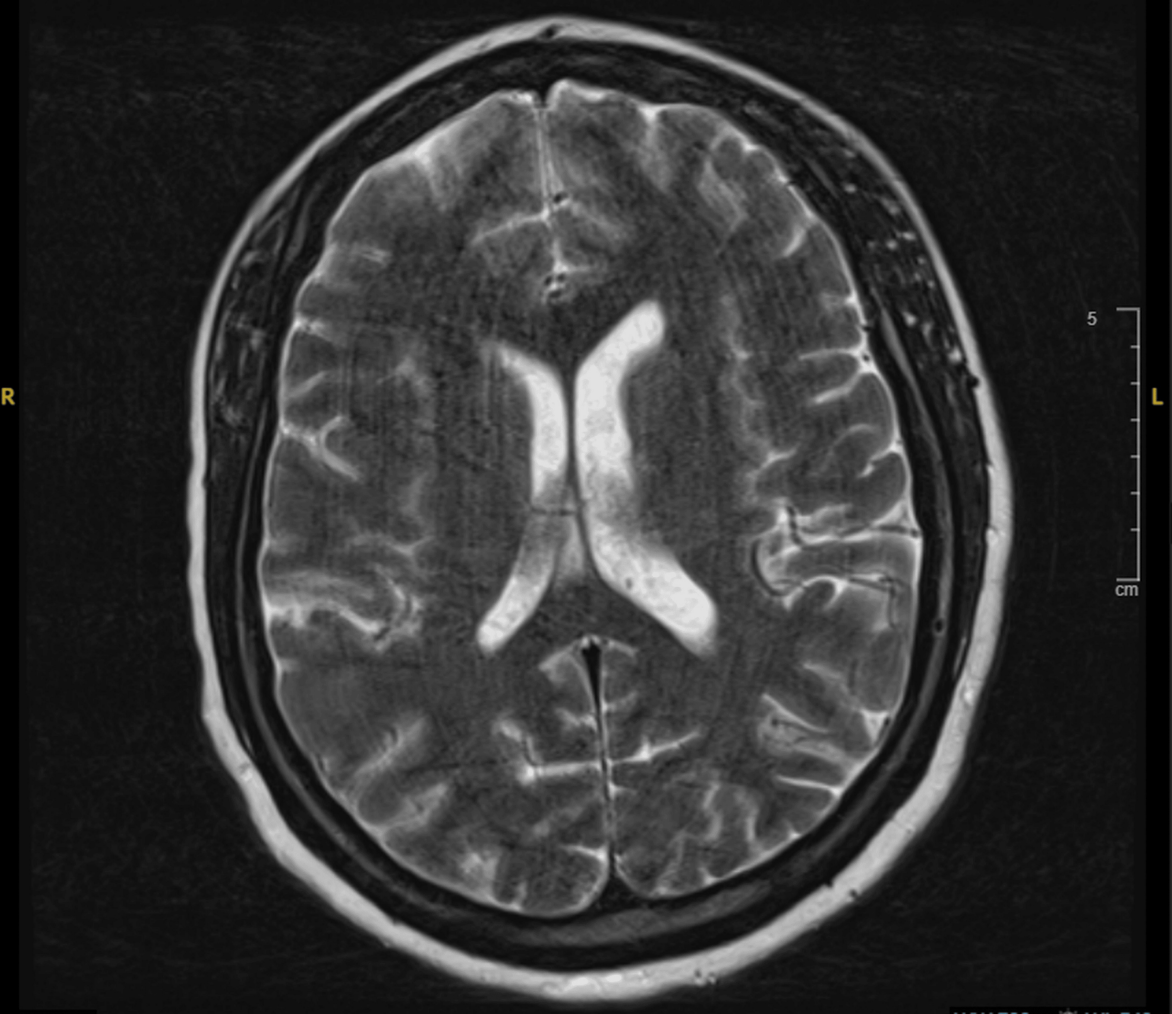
MRI brain showing no acute intracranial findings.
